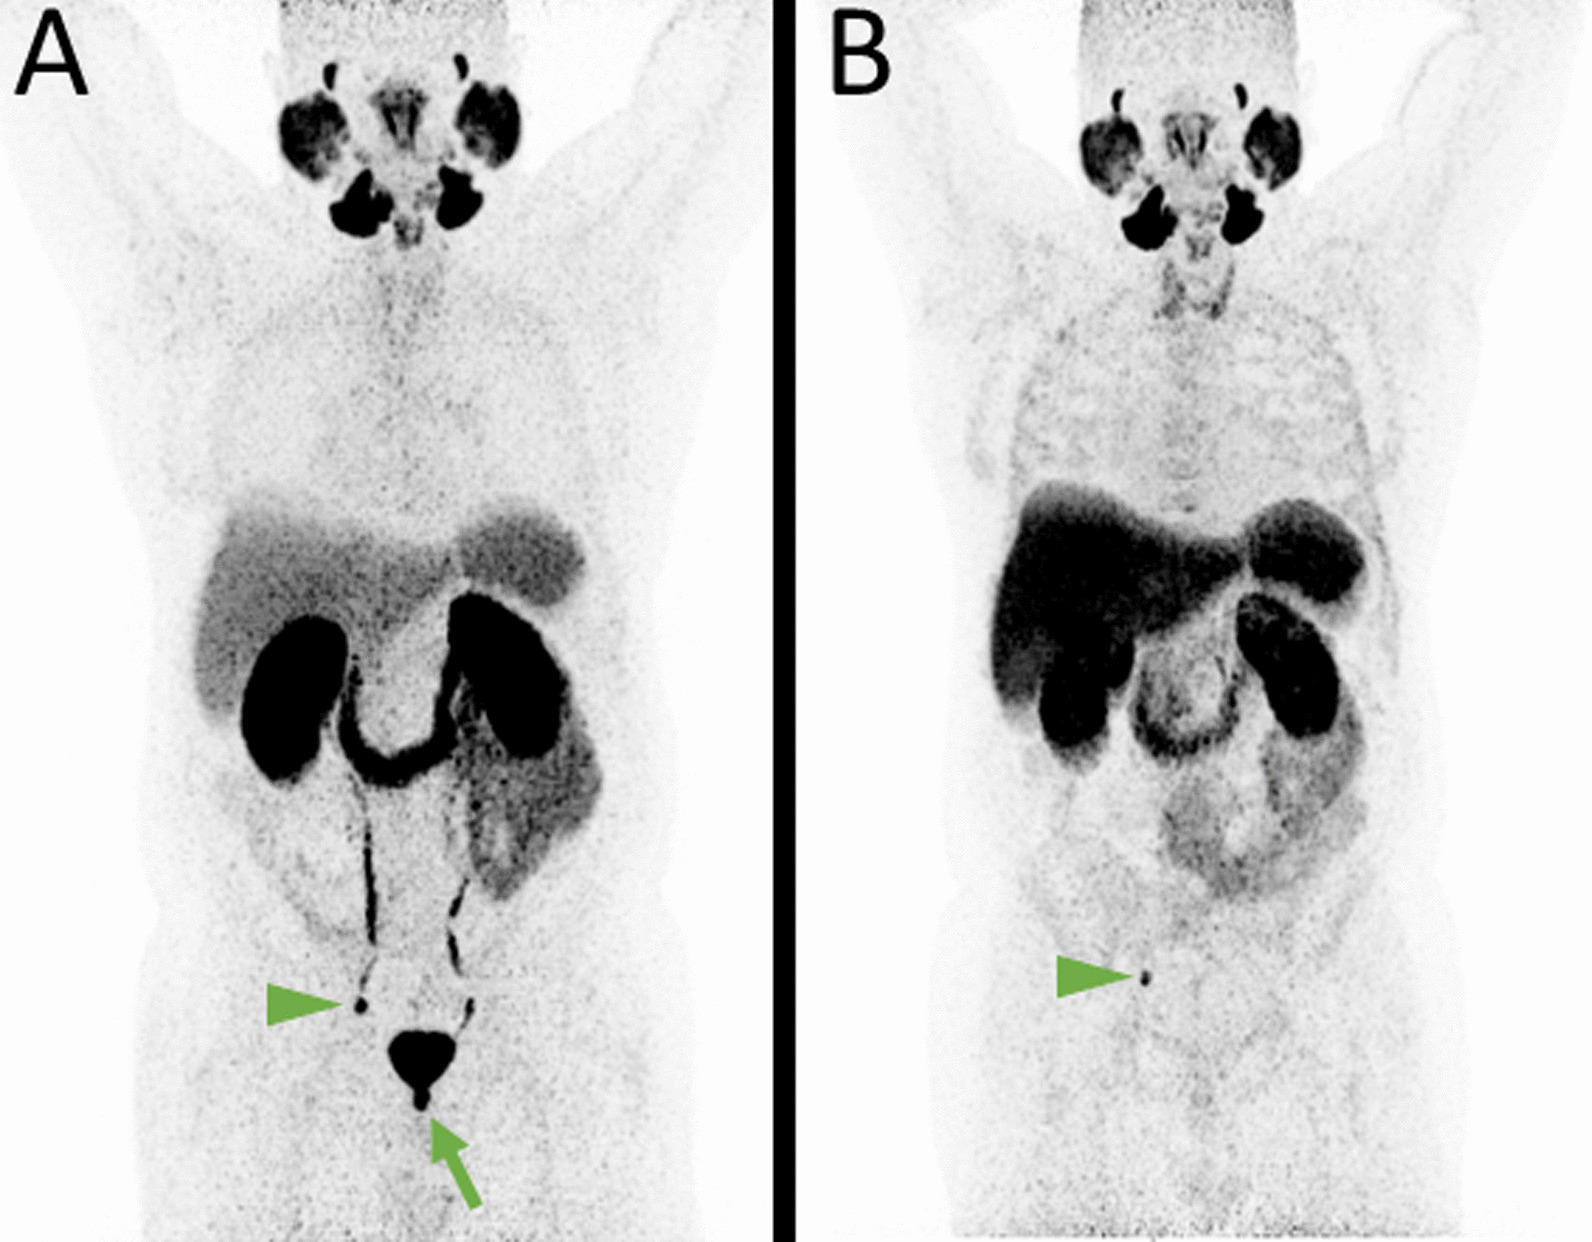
MIP of [^68^Ga]-Ga-PSMA-11 (A) and [^18^F]-F-PSMA-1007 PET (B). Only in the [^68^Ga]-Ga-PSMA-11 PET, intense uptake in the prostatic fossa is noteable (green arrow). The absence of any uptake in [^18^F]-F-PSMA-1007 PET suggests the presence of urinary retention. Concordantly present external iliac lymph node metastasis on the right (arrow heads). However, in [^18^F]-F-PSMA-1007 PET due to missing ureteral activity, the demarcation is much better. Auxiliary finding in B Disseminated presumable unspecific uptake in the skeleton and moderate thyreoidal uptake
